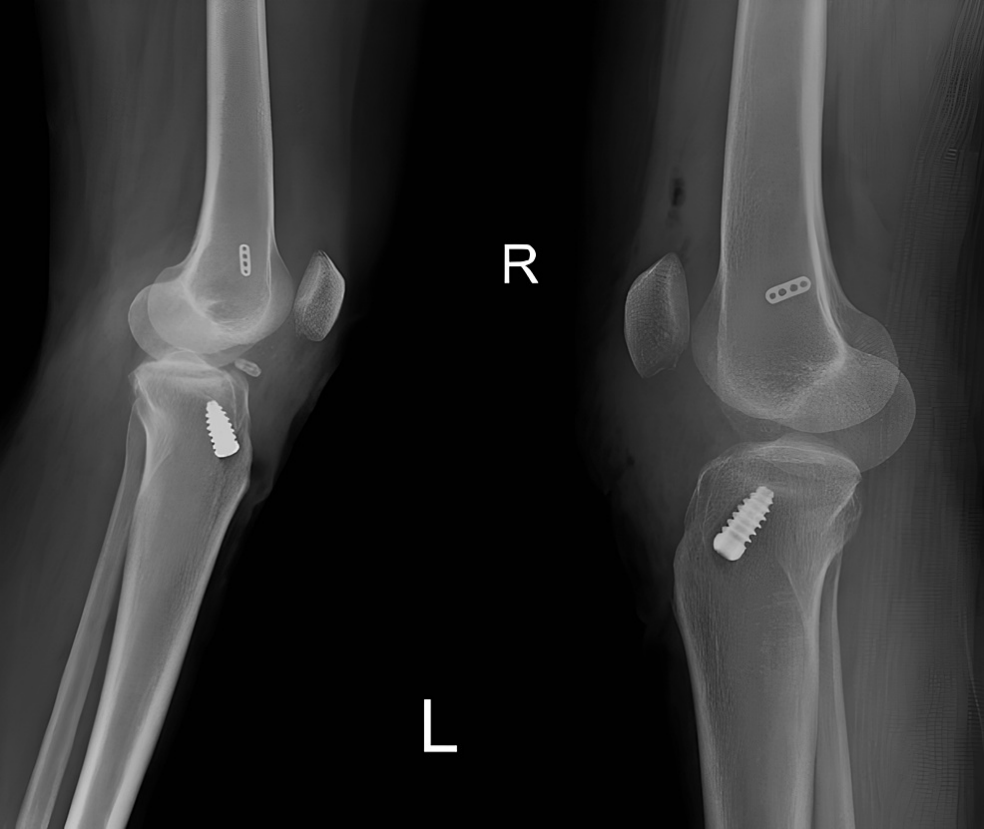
Post-operative X-ray bilateral knee lateral view
